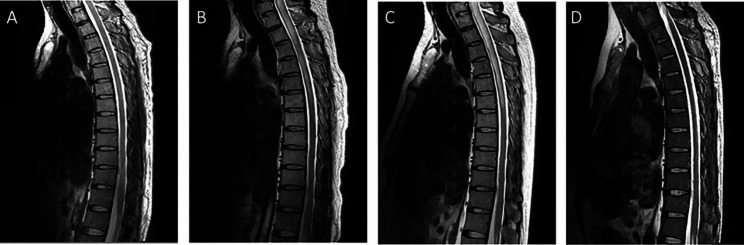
Serial sagittal MR images at presentation and follow-up. Initial sagittal image (A) revealed no obvious abnormality in the thoracic spinal cord. Repeated MRI after 1.5 months (B) showed the swelling of the thoracic cord and cord-like abnormal T2 signal. MRI at 2 months from presentation (C) showed similar lesion. MRI at 6 months from presentation (D) revealed the lesion significantly reduced
